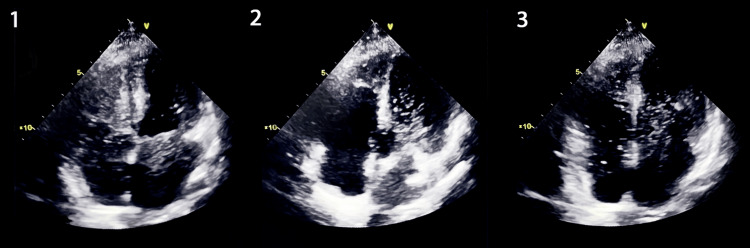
Contrast saline transthoracic echocardiography of the first patient in three phases. Sequence 1 revealing micro-bubbles in the right chambers at the moment of saline injection; sequences 2 and 3 revealing micro-bubbles in the left chambers after the sixth and seventh cardiac cycles, respectively
